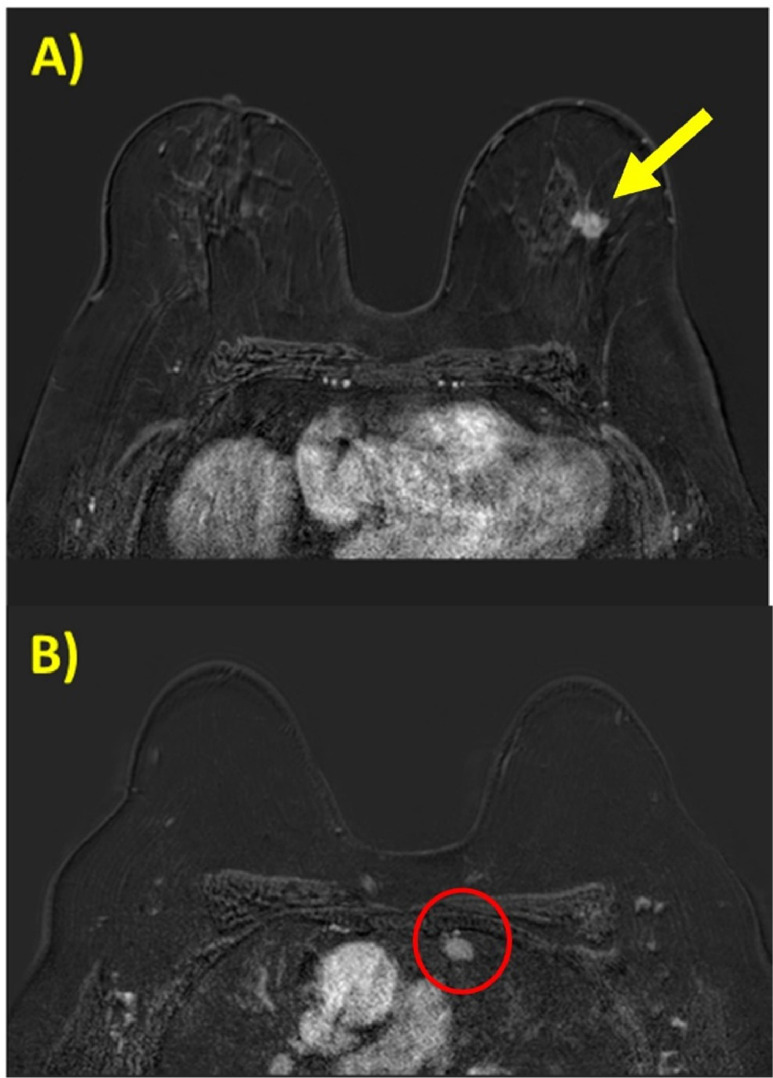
(A and B) Axial T1-weighted 3D acquisition of the first post-contrast dynamic frame showed: (A) an irregular lesion with heterogeneous mass enhancement in the upper outer quadrant of the left breast (yellow arrow); (B) a round-shaped formation with homogeneous contrast enhancement in the left side of the upper-anterior mediastinal area (red circle).
